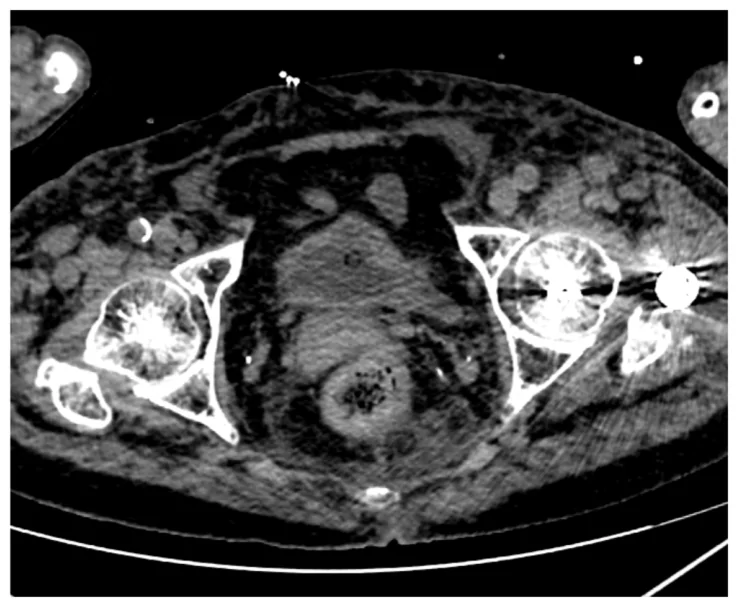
Non-contrast CT of the whole abdomen on admission showed no evidence of intra-abdominal hemorrhage or hematoma.
